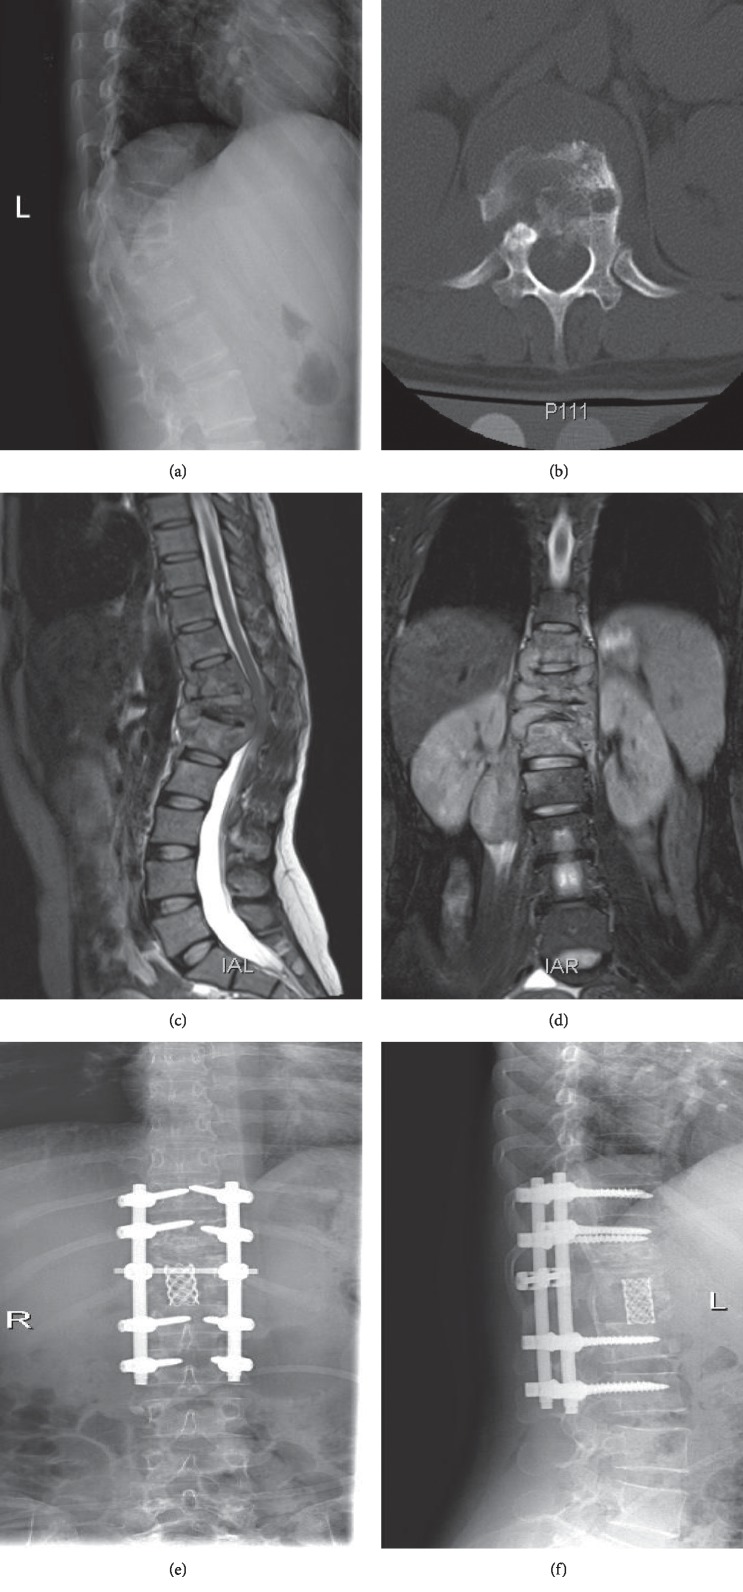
(a) Radiograph of a 32-year-old woman showing T9-L2 vertebral destruction with kyphosis. (b) Axial CT scan showing T12 vertebral destruction. (c) Sagittal MRI showing T9-L2 vertebral destruction with kyphosis, paravertebral, and intraspinal abscesses. (d) Coronal MRI showing T9-L2 vertebral destruction with paravertebral and psoas abscesses. (e, f) A postoperative radiograph shows deformity corrected and satisfactory positioning of the internal fixation device.
